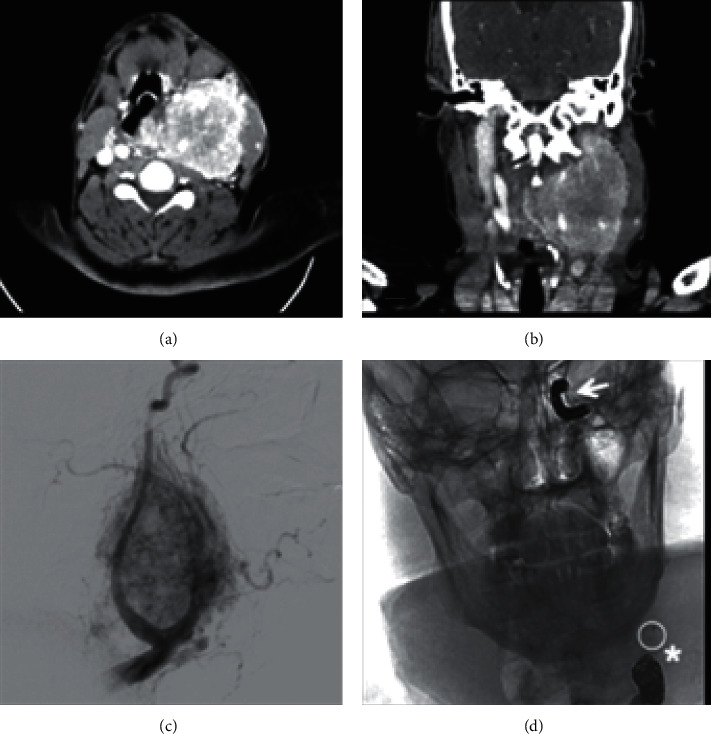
(a) An axial CT image showing the complete engulfment of the inner and outer carotid arteries by the tumor. (b) A reconstructed coronal image showing the tumor's extension to the skull base. (c) An angiogram showing splaying of the inner and outer carotid vessels (lyres sign) and tumor blush. (d) A postembolization picture showing the coils in the left common carotid artery (star) and in the left internal carotid artery above the skull base (arrow), and the vascular plug in the left external carotid artery (circle).
